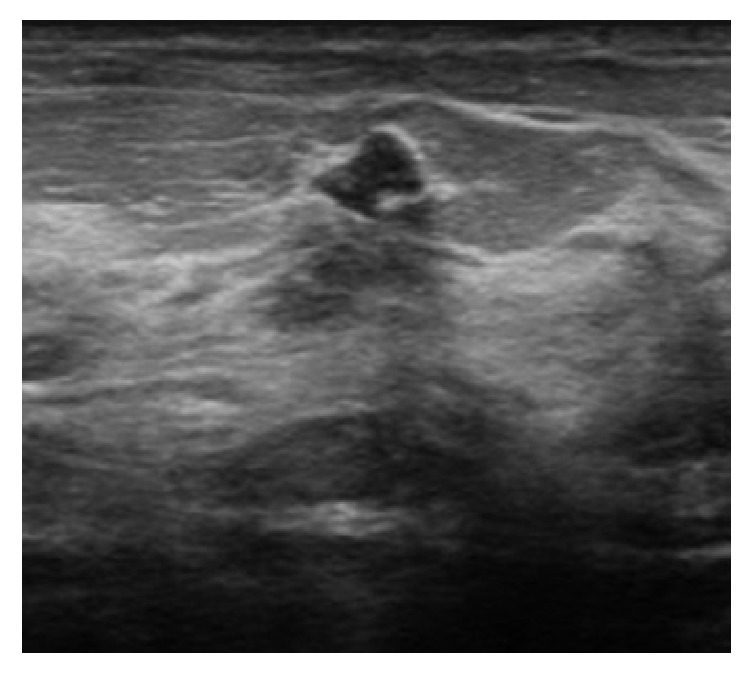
40-year-old woman presenting for screening mammography and ultrasound. Mammography (not shown) revealed dense breasts and no focal lesions. Ultrasound showed a hypoechoic mass with angular margins. Biopsy shows stromal fibrosis. Follow-up after two years showed no malignancy.
